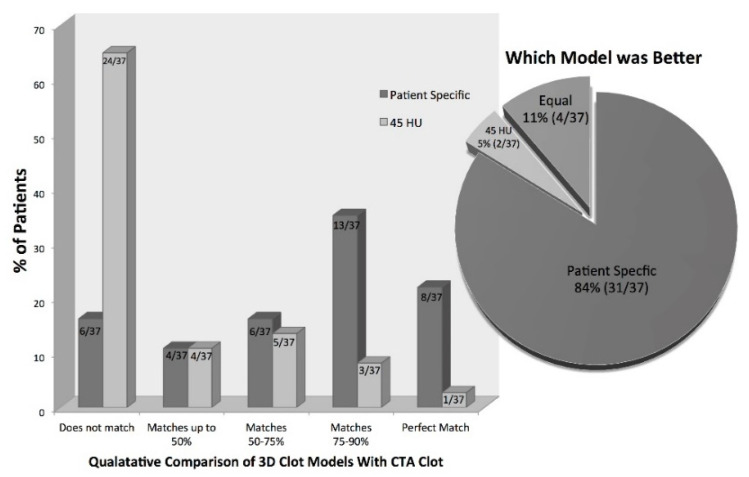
Qualitatively comparing three-dimensional models of the hyperdense thrombus in NCCT with CTA thrombus. Three-dimensional model formed using either (1) calculated patient-specific thresholds or (2) conventional single 45HU threshold.
